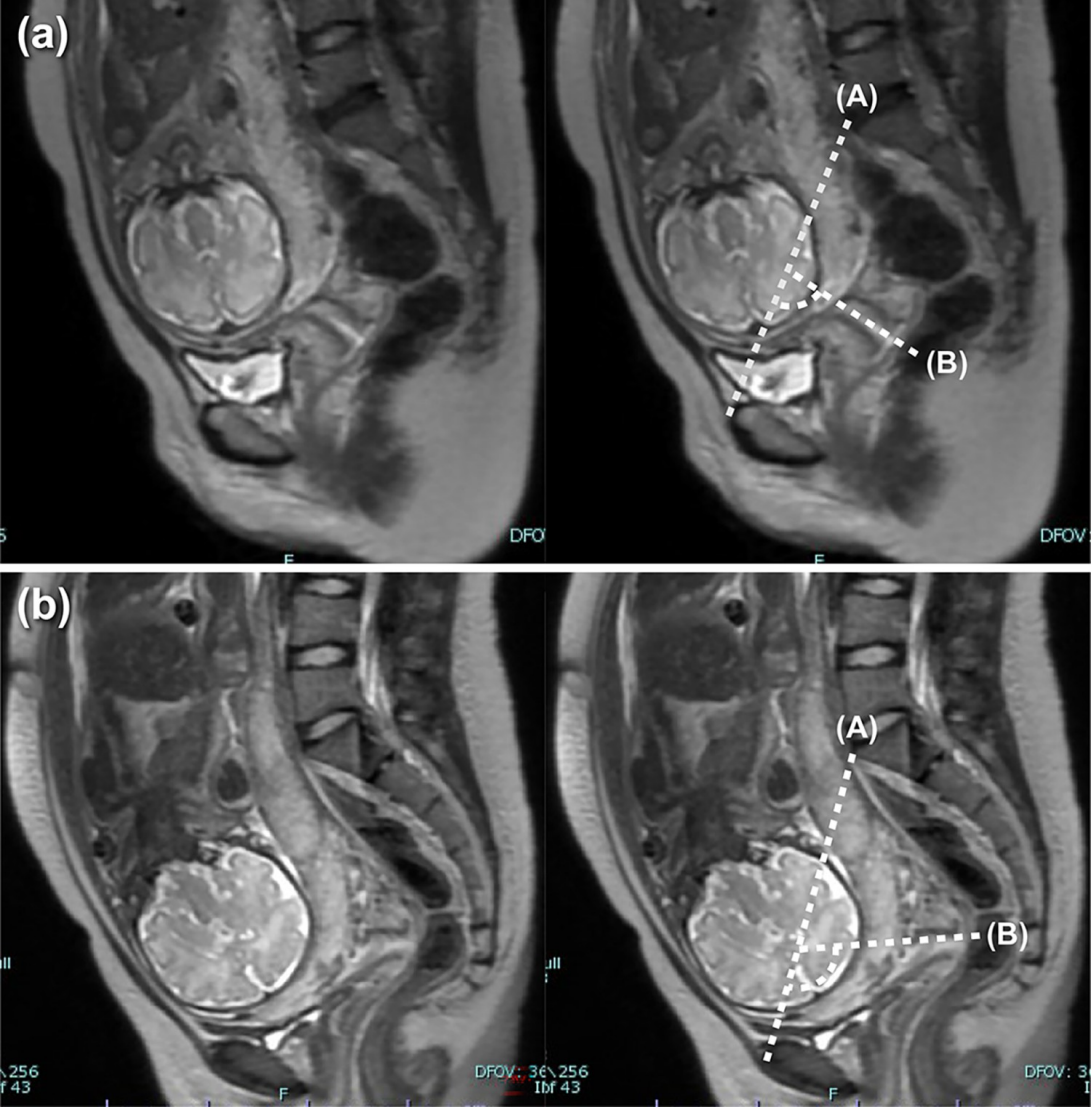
Magnetic resonance imaging (MRI) showing measurement of the cervical inclination angle. (a) Typical MRI findings in a patient without pouch of Douglas obliteration. (b) Typical MRI findings in a patient with pouch of Douglas obliteration.
